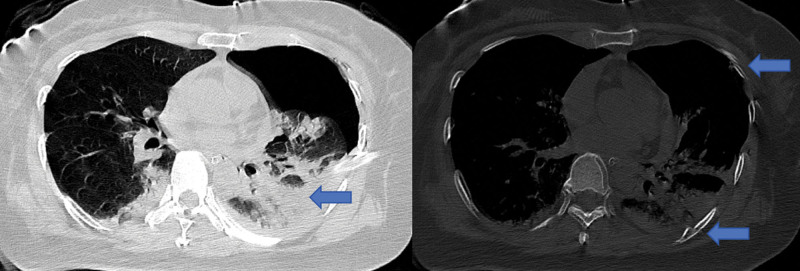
Thoracic CT findings. Contrast-enhanced thoracic computed tomography (CT) images showing left-sided hydropericardium (solid arrow), haemopneumothorax (asterisk), pulmonary contusion (arrowheads), and multiple rib fractures (circles). These findings indicate major blunt thoracic trauma with active intrathoracic bleeding. CT = computed tomography.
